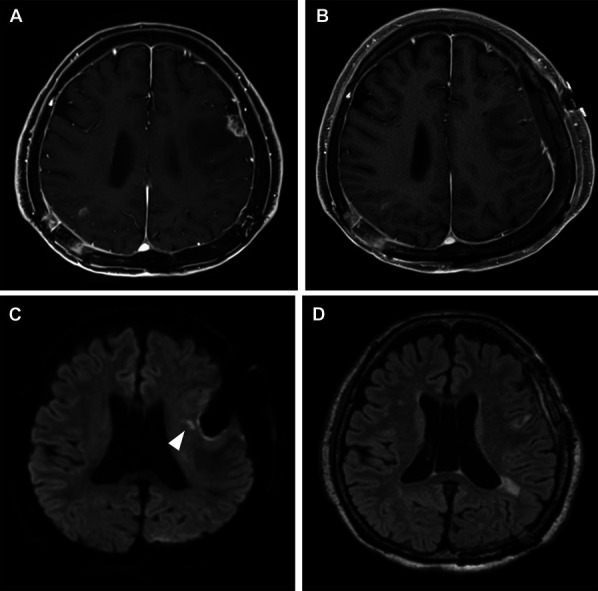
A, MRI of a 73-year-old man after craniotomy and stereotactic radio surgery showing recurrent brain metastasis. B, Awake craniotomy to achieve gross total resection is performed. Fifteen hours after surgery, motor aphasia appeared. C, DWI-MRI showing cerebral ischemia in the deep white matter (arrowhead) without a high-intensity area on D, the fluid-attenuated inversion recovery image corresponding to the ischemic lesion.
